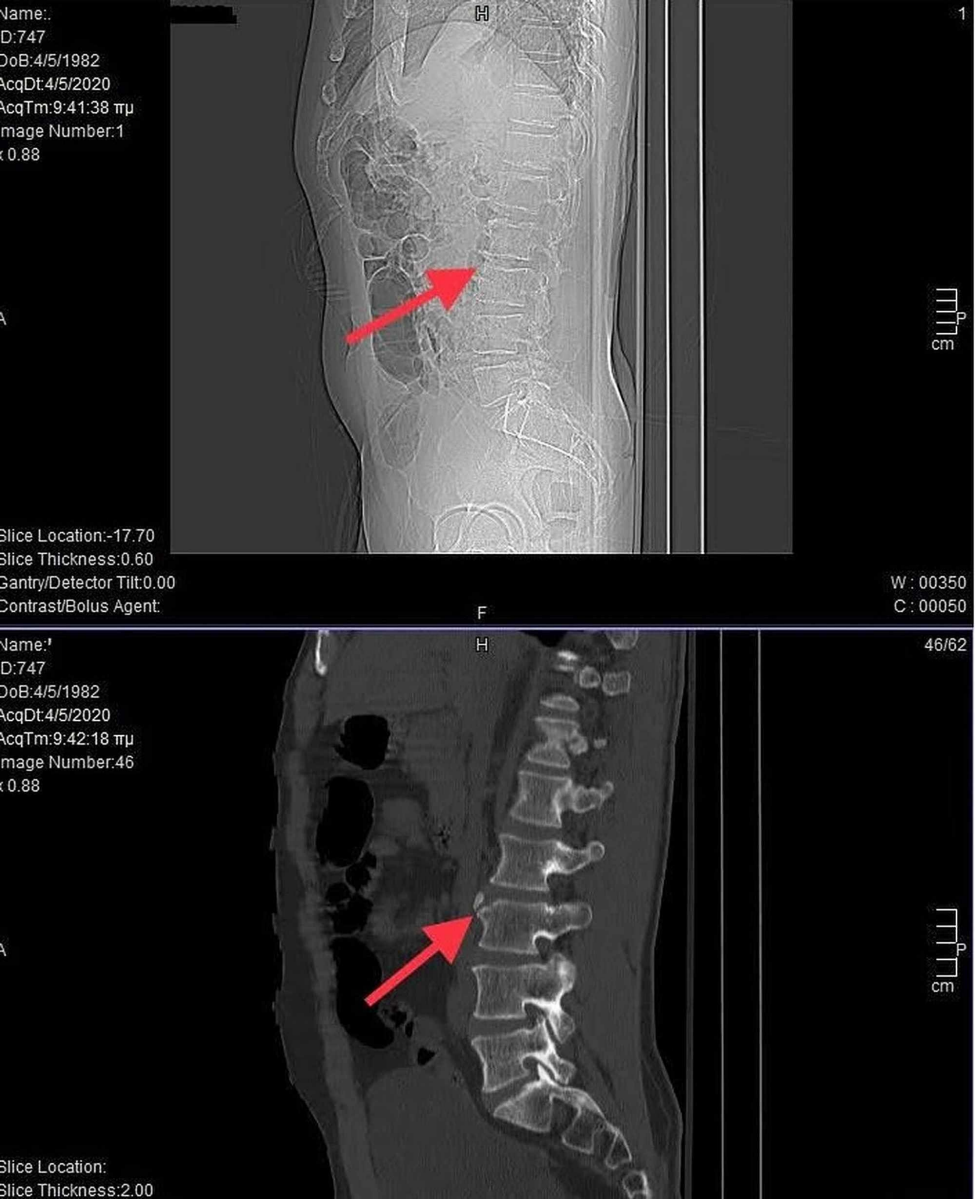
CT scan of lumbar spine showcasing a detached sclerotic triangular bony fragment on the anterior aspect of L3 superior endplate.
